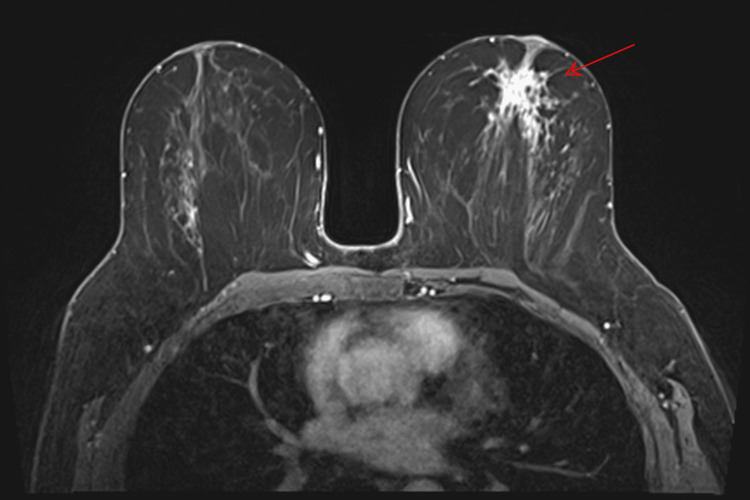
Breast MRI Non-mass enhancement in the upper retroareolar region of the left breast, with segmental distribution and spiculated margins, measuring 3.8 × 2.5 × 1.9 cm (red arrow). MRI: magnetic resonance imaging
